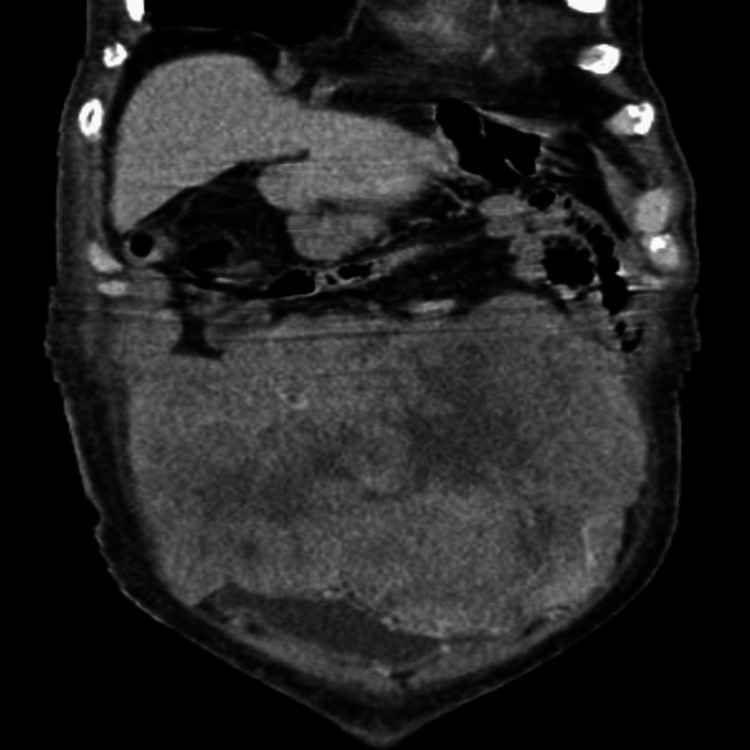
CT abdomen showing a large abdominopelvic mass lesion
